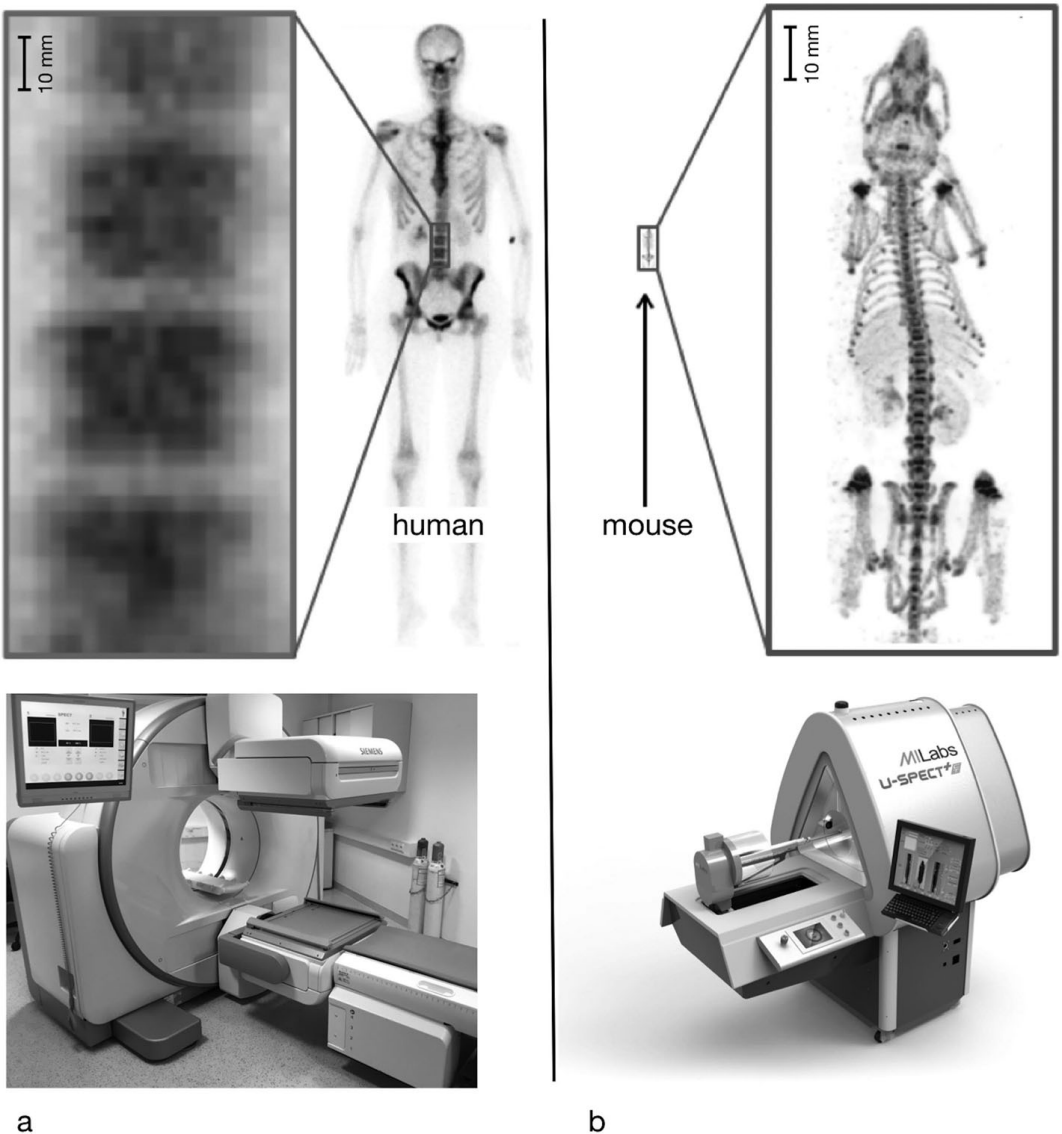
Spatial resolution performance comparison in SPECT between a clinical (a) system and a preclinical system (courtesy of MILabs B.V) (Ivashchenko et al. 2015) (b)
